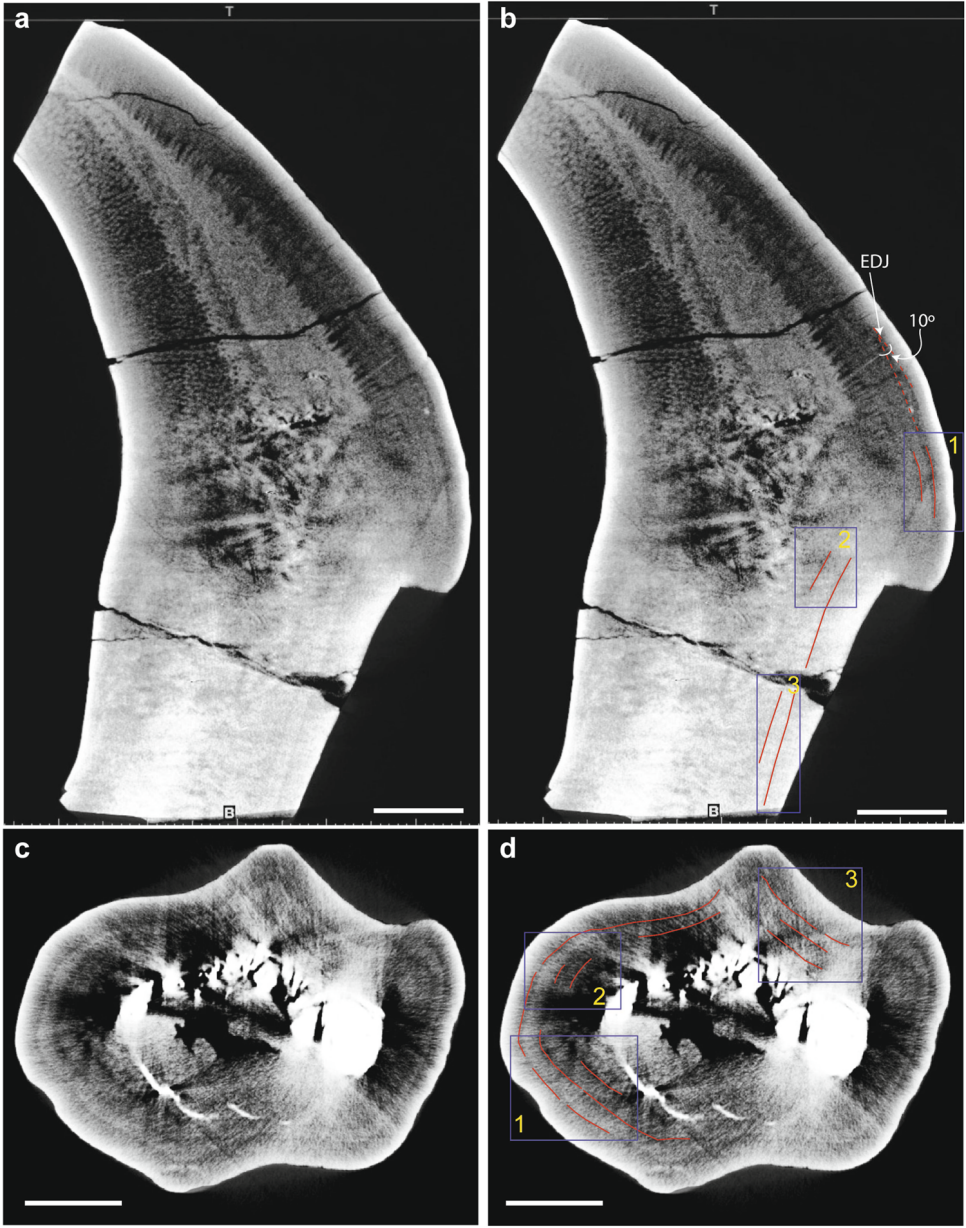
CT images of dentary tooth in (a) coronal view, (b) coronal view with incremental lines measured in three regions. Measured angle of the incremental line relative to the enamel-dentine juncture (EDJ) is ~10° (c) axial view and (d) axial view with incremental lines measured in three regions. Scale bar = 1 cm.
